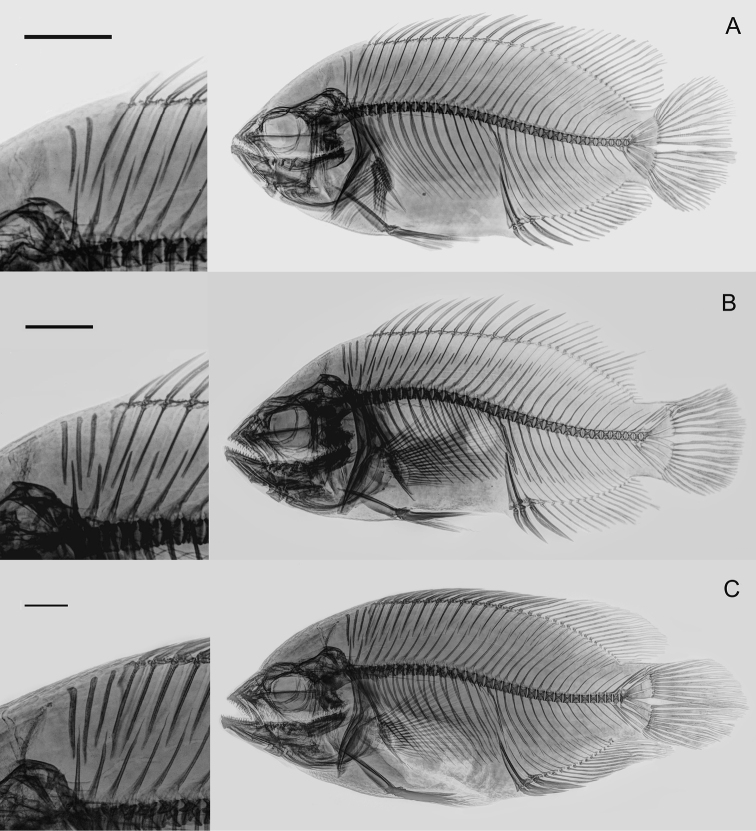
Radiographs of paratypes of *Astronotusmikoljii* sp. nov. and details of the supraneural bones AMHNLS 198 (116.7 mm SL) BMHNLS 26123 (140.4 mm SL) CMHNLS 24054 (227.5 mm SL). Scale bars: 10 mm.
